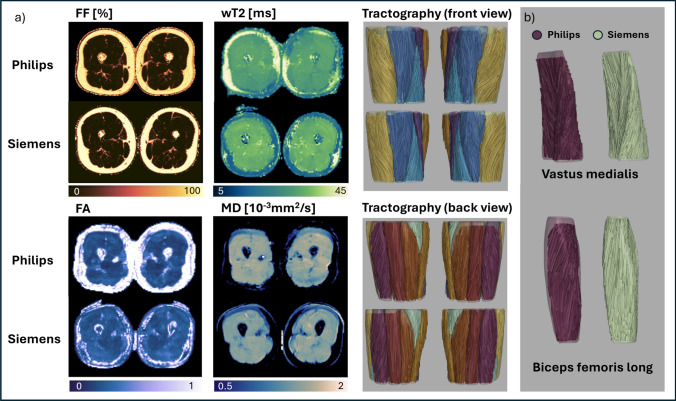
a Overview processed imaging data from a representative subject, showing quantitative MRI parameters, fat fraction (FF), water T2 relaxation time (wT2), fractional anisotropy (FA), mean diffusivity (MD) as well as diffusion tractography for upper leg muscles Color coding: yellow—vastus lateralis; dark blue—rectus femoris; light blue—vastus medialis; dark purple—gracilis; gold—sartorius; purple—biceps femoris long head; red—semitendinosus; orange—semimembranosus; turquoise—adductor magnus. b tractography of two representative muscles
